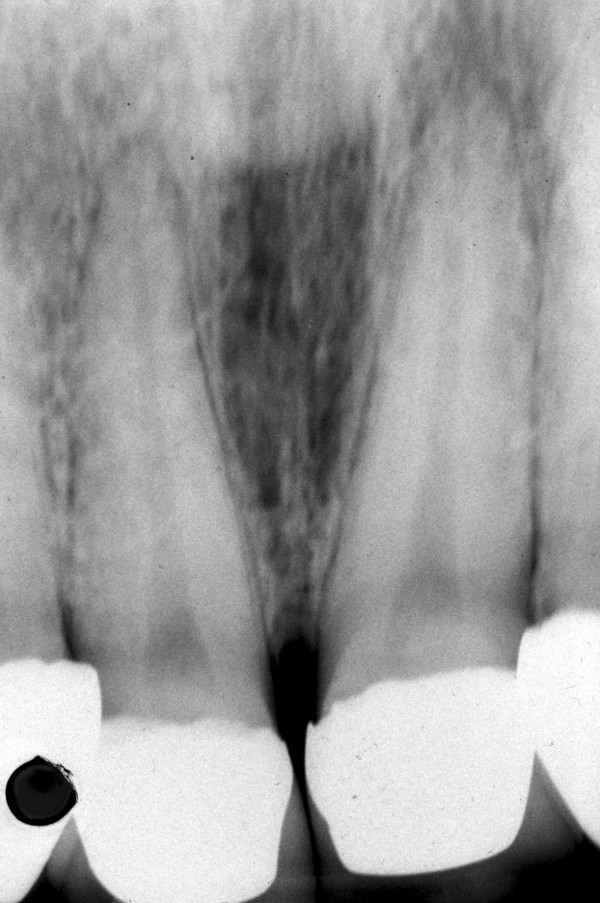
Intra-oral radiograph indicating the crown margins in relation to the cemento-enamel junction.
